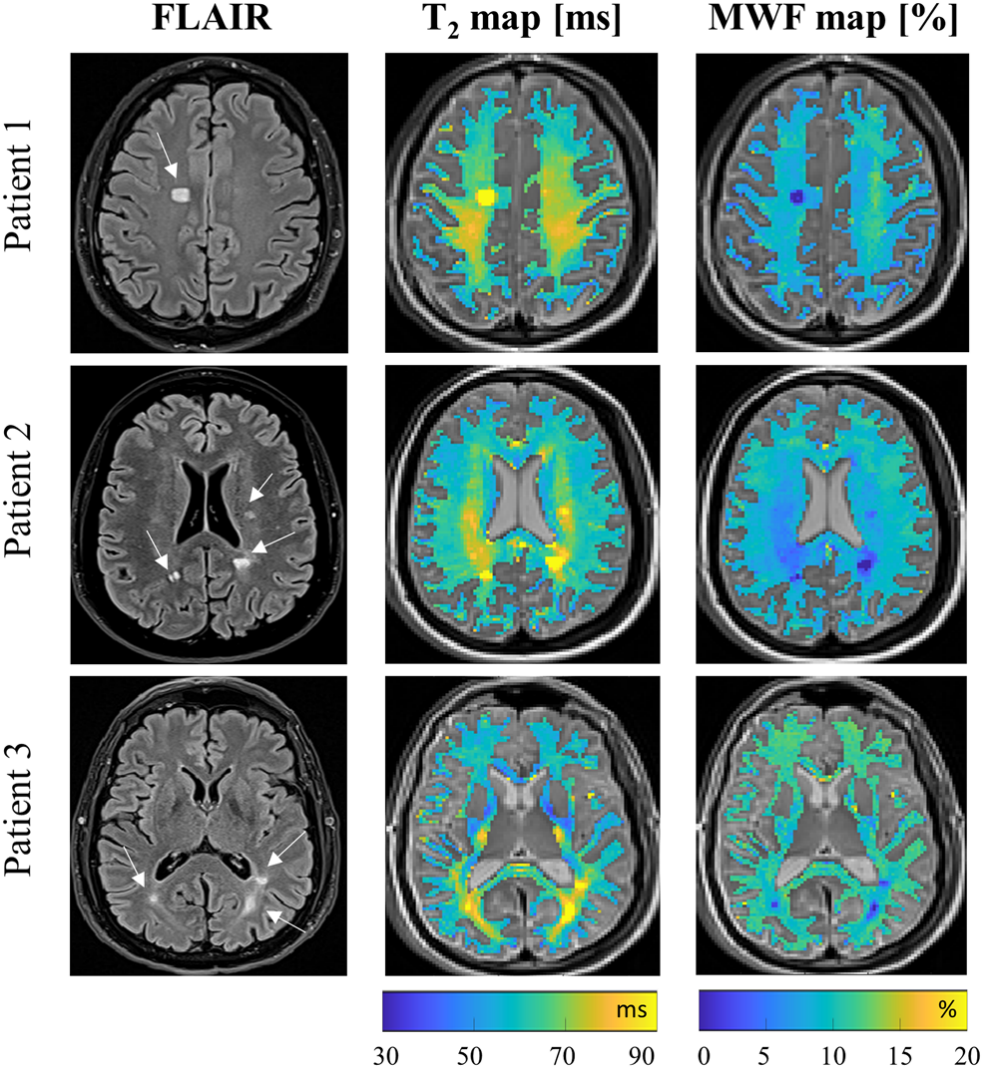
FLAIR images, T_2_maps, and MWF maps for three people with MS fitted using the data-driven approach.
